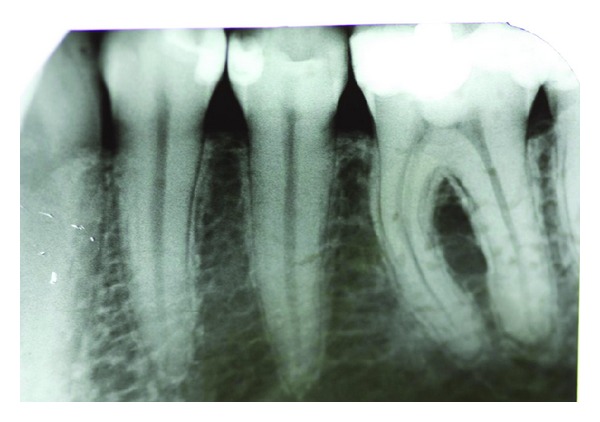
Radiolucency image of tooth 36 showing a periapical radiolucency in the region of the mesial and distal root apices.
